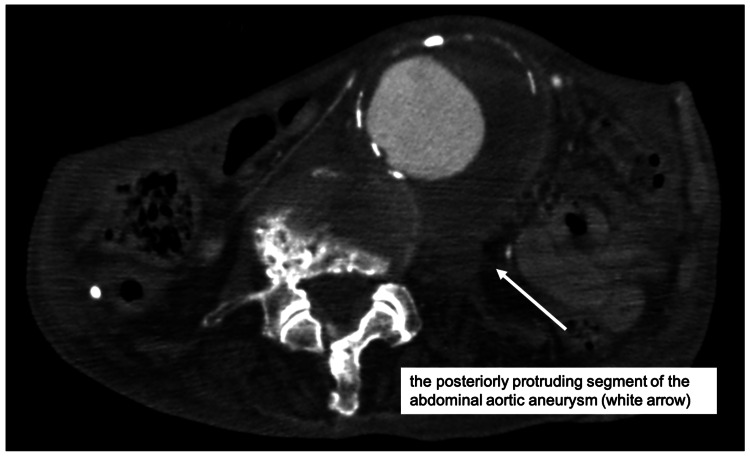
Computed tomography angiography showed an abdominal aortic aneurysm that was enlarged and protruding posteriorly.
